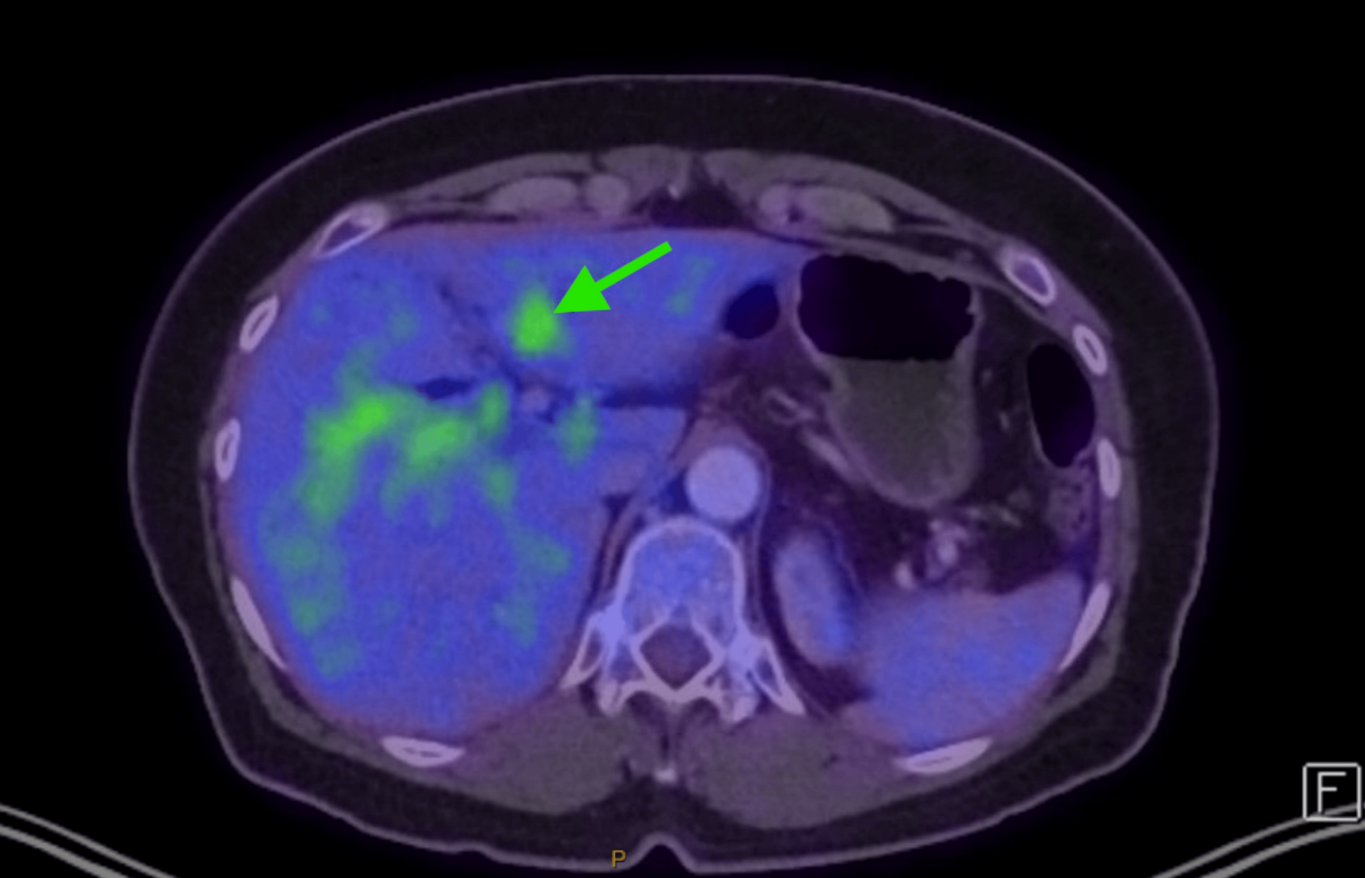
PET demonstrated a focal area of increased FDG uptake in the left lobe of the liver, which correlated closely with a hypoenhancing lesion on MRI and, therefore, was very suspicious for malignancy Green arrow: FDG uptake in the left lobe of the liver PET: positron emission tomography; FDG: fluorodeoxyglucose
